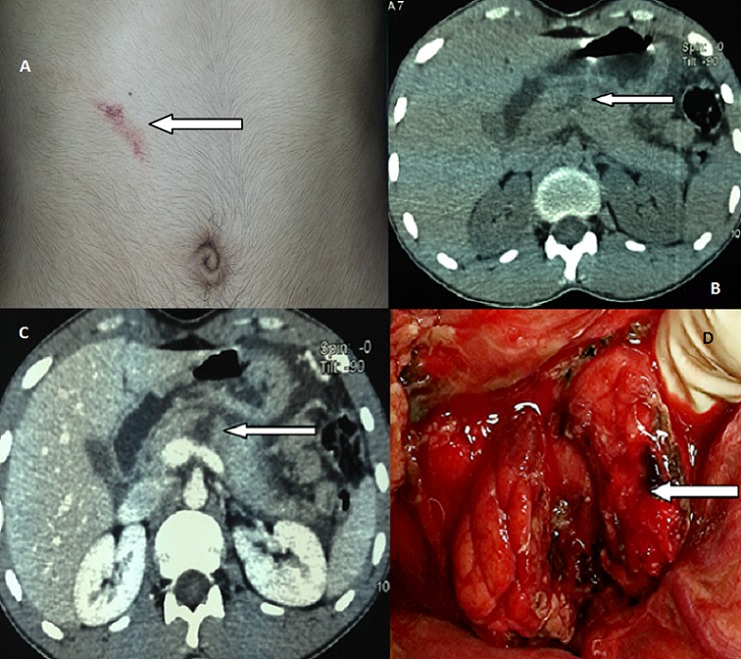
A) siège du traumatisme abdominale Clinical picture showing the seat of abdominal trauma; B) tomodensitometries montrant une fracture isthmique du pancreas CT image showing a fracture of the isthmus pancreas; C) tomodensitometriques montrant une fracture isthmique du pancreas CT image showing a fracture of the isthmus pancreas; D) Image per opératoire montrant une fracture pancréatique Intraoperative picture showing a pancreatic fracture
